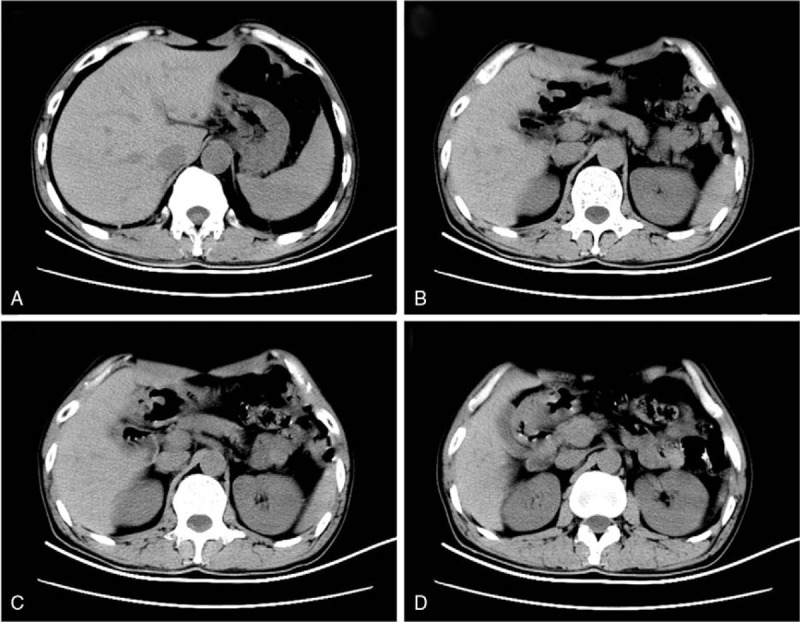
Abdominal computed tomography revealed a thickened gastric wall in the antrum.
